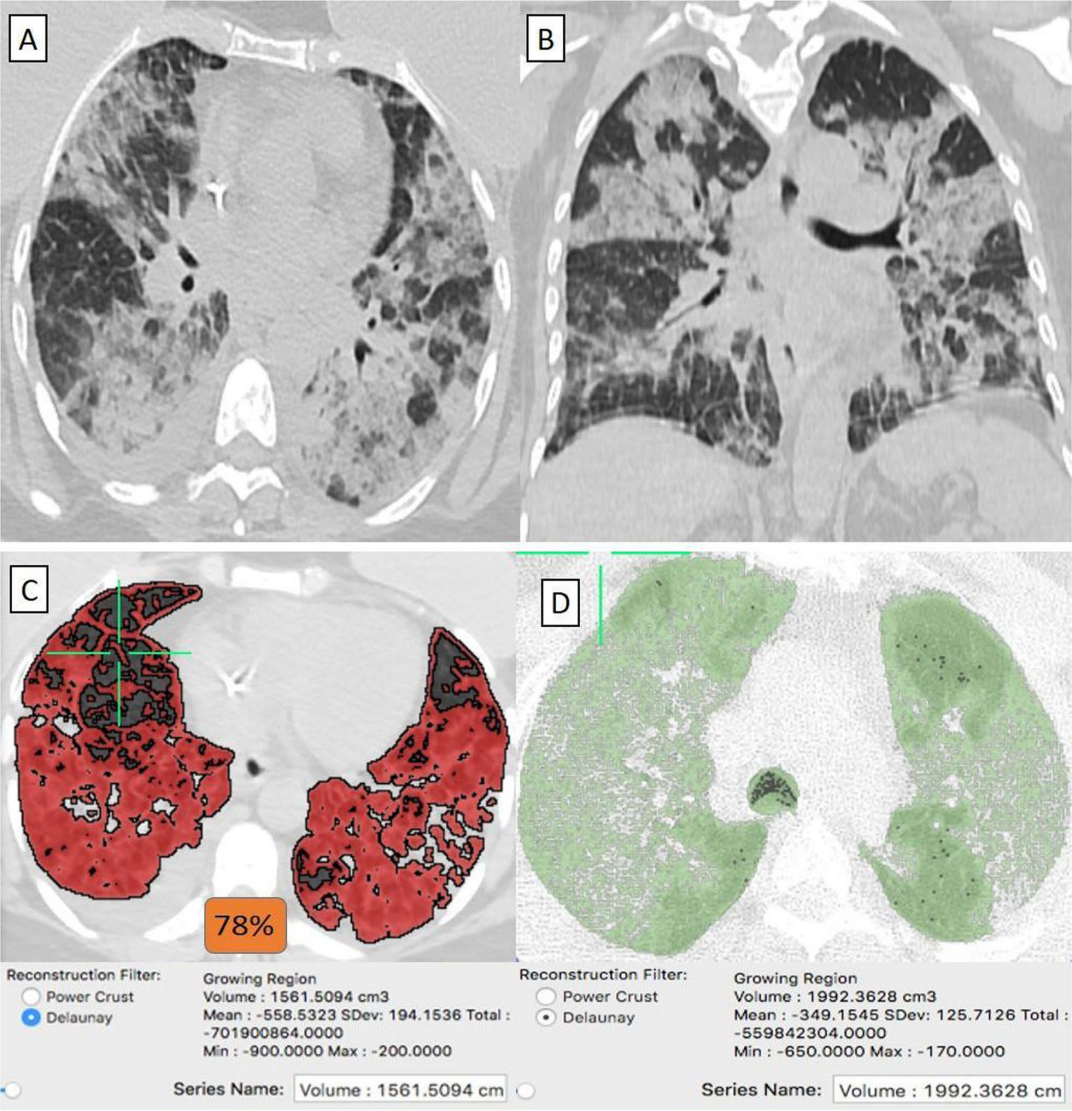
A 53-year-old female patient with history of operated bilateral breast cancer and had received “Sinopharm” vaccine then got COVID-19 infection. A Axial, and B Coronal chest CT cuts (lung window) showed bilateral wide spread large heterogeneous ground-glass patches with consolidative changes and minimal right pleural collection. Breast implants are also noticed in axial cuts. C CT-Volumetry of the pathological lung parts averages 1561 cc. D CT-Volumetry of total lung volume averages 1992 cc. So, 78% of the lung parenchyma was diseased (Score 4)
